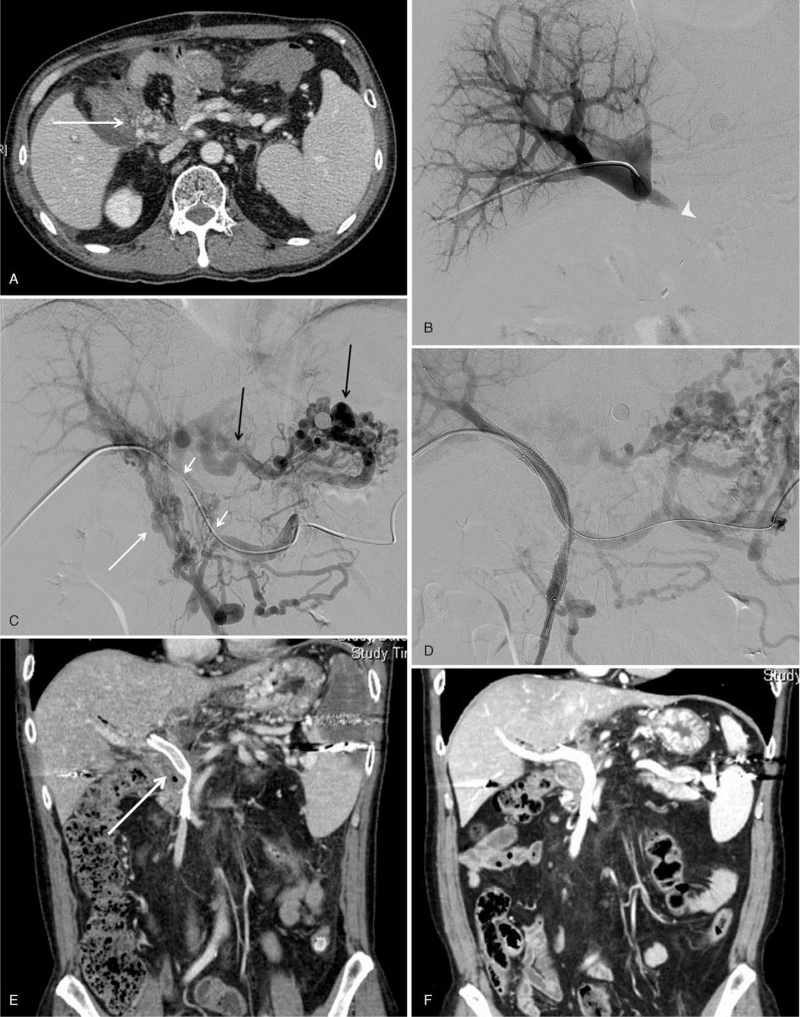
A 45-year-old male patient who underwent portal vein stenting due to recurrent hematochezia after 56 months of pylorus-preserving pancreaticoduodectomy for pancreatic cancer. (A) CT image showing varix in afferent jejunal loop (white arrow). (B) Main portal vein occlusion detected by portal venography via transhepatic approach (white arrowhead). (C) Segmental occlusion of main portal vein (short white arrows), jejunal varix connected to the right portal vein by forming a complex and extensive collateral around the afferent jejunal loop (long white arrow) and gastric varix with posterior gastric vein dilatation connected to the left portal vein (long black arrows). (D) Recanalization from SMV to MPV without jejunal collateral vessel after stenting (9 mm in diameter and 80 mm in length). (E) Improvement of varix of jejunal limb (long white arrow) confirmed on follow-up CT on the 8th day after stenting. (F) Patent stent graft shown on CT performed 78 months after stenting.
